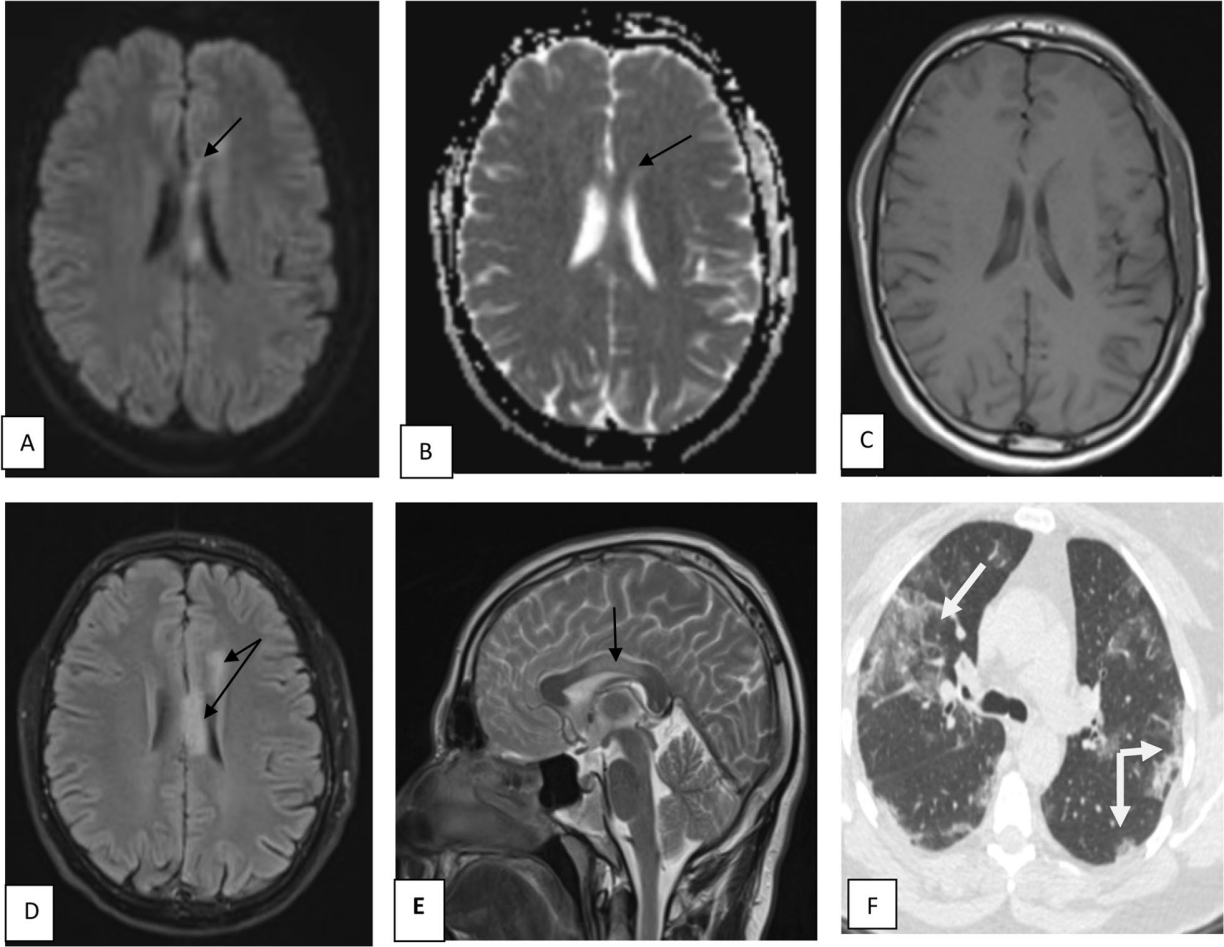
Male patient aged 29 years old, with history of COVID-19 infection 1 month ago, presented by headache, weakness in right side of the body and tingling started 3 days ago. MRI brain showed well-defined callosal and pericallosal left frontal area of altered signal intensity (black arrows) denoting subacute infarction, displaying high signal intensity in DWI (A) and intermediate signal intensity in ADC map (B) denoting free diffusion. The lesion displays isointense signal in T1WI (C) and high signal intensity in FLAIR sequence (D) and T2WI (E). Axial CT chest cut (F) of the same patient shows bilateral peripheral ground-glass opacities (white arrows) (moderate severity)
